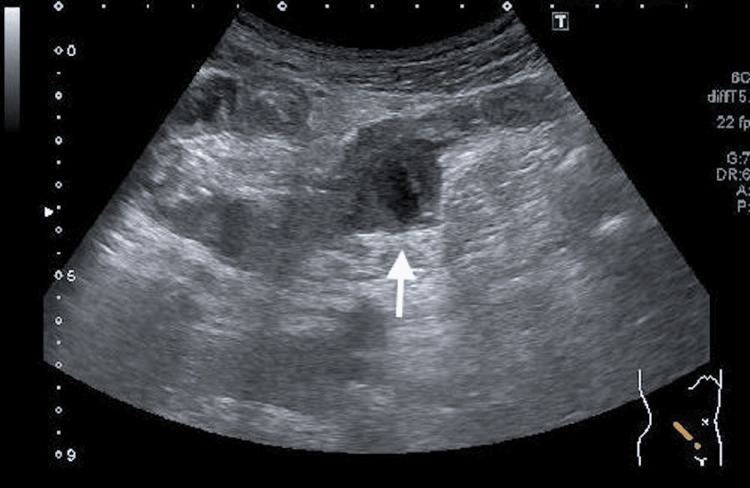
Abscess formation at the site of diverticulitis observed on the plane CT (arrow)
